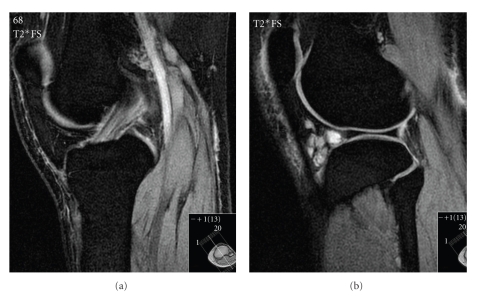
Para-sagittal and sagittal T2 FS MRI scans confirming a normal ACL signal and extensive extension of cystic lesion into Hoffa's fat pad.
